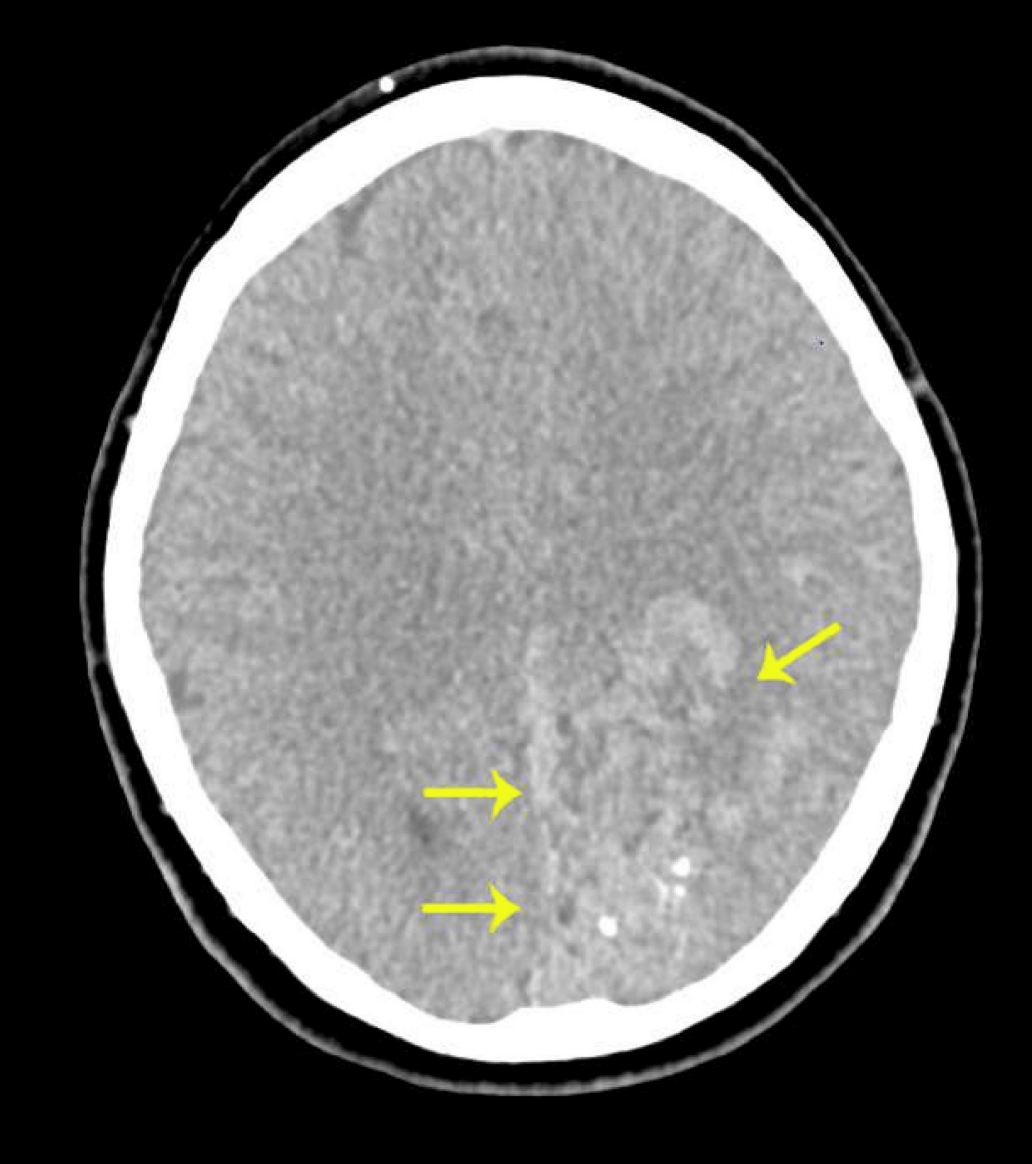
Non-contrast computed tomography (CT) of the head showing multiple curvilinear hyperdensities and punctate calcifications in the left parieto-occipital and temporal lobes. Surrounding vasogenic oedema and a 3 mm rightward midline shift are noted, without any evidence of acute haemorrhage.
